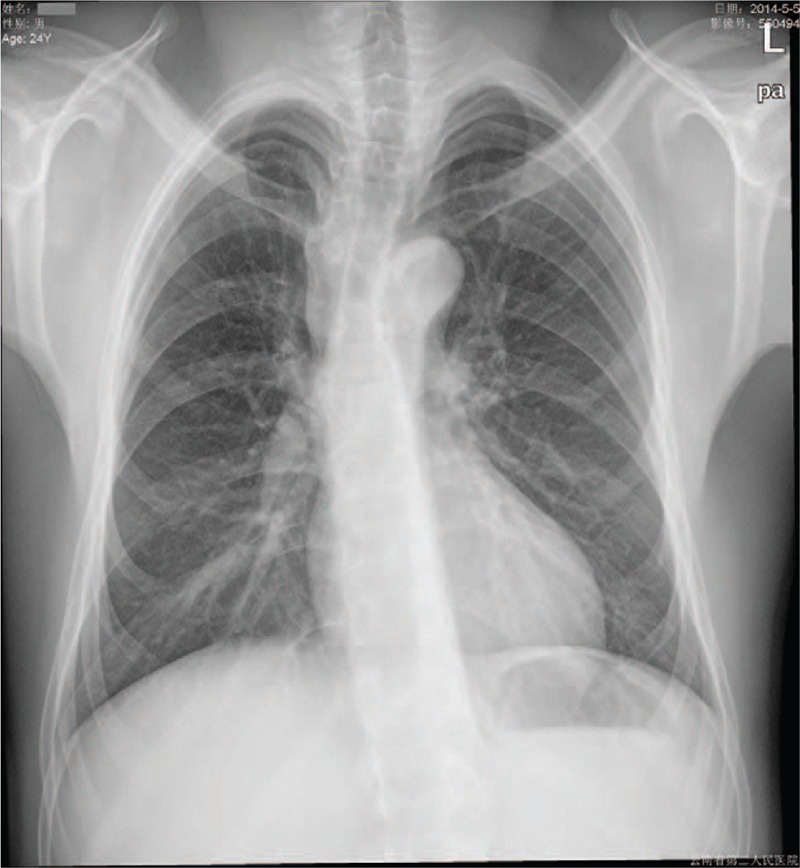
Chest X-ray film showing scoliosis, mild platspondyly, and osteoporosis of thoracic vertebral body.
